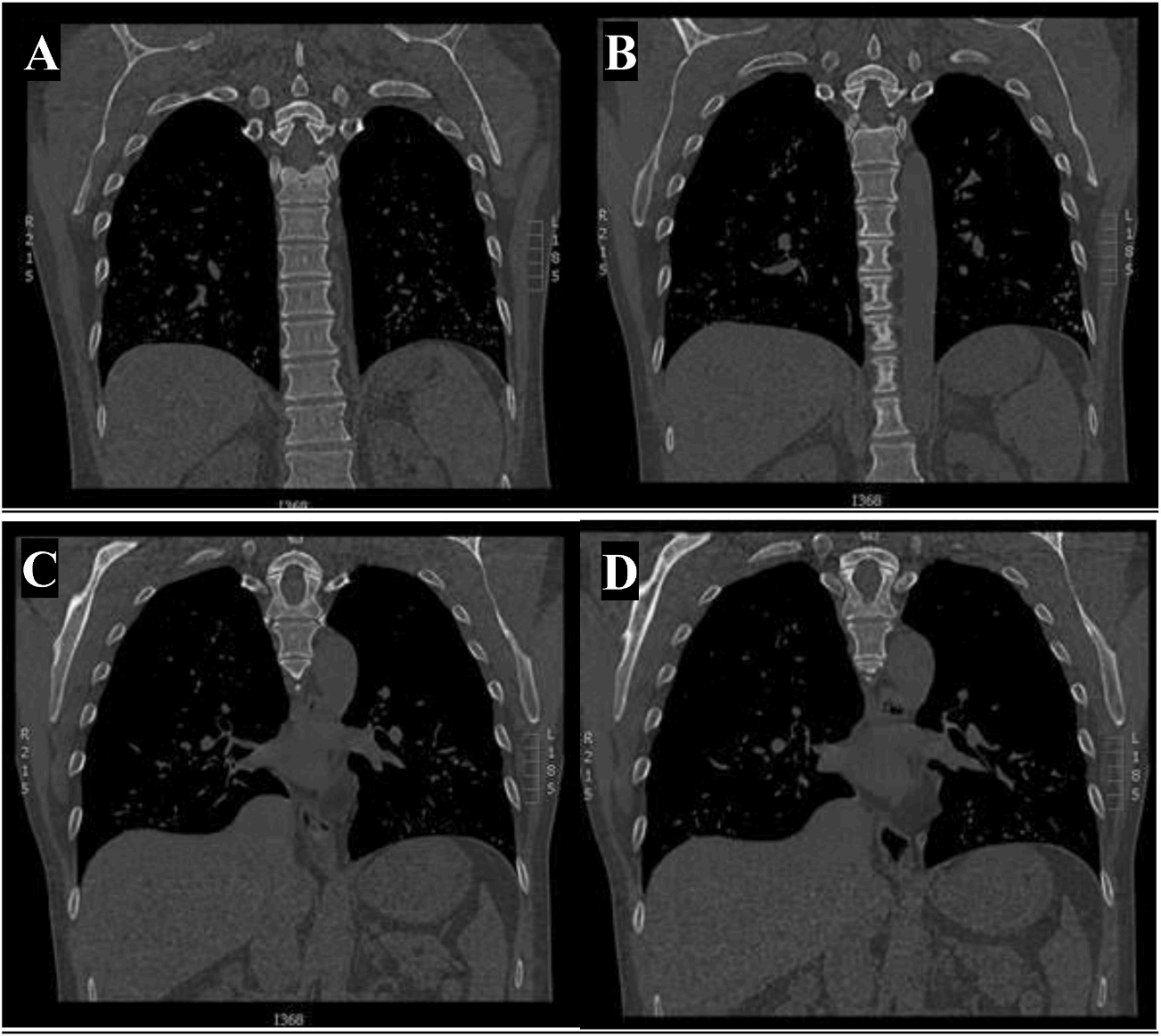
Four different computed tomography coronal cut bone windows.
